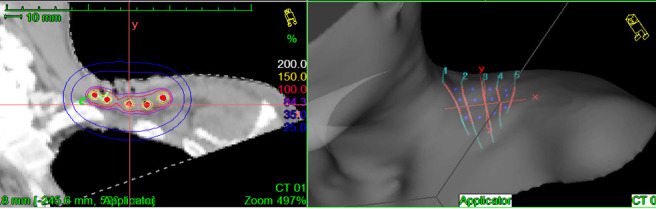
CT cross section image showing brachytherapy catheters with dose distribution
